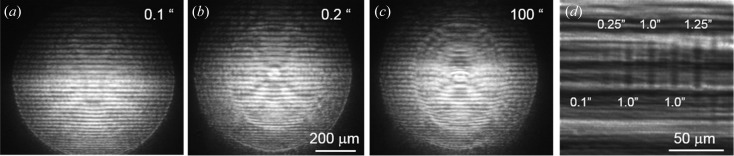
(*a*)–(*c*) Experimental reflecto-interferograms recorded at different exposures. (*d*) X-ray topogram of the damaged structure.
